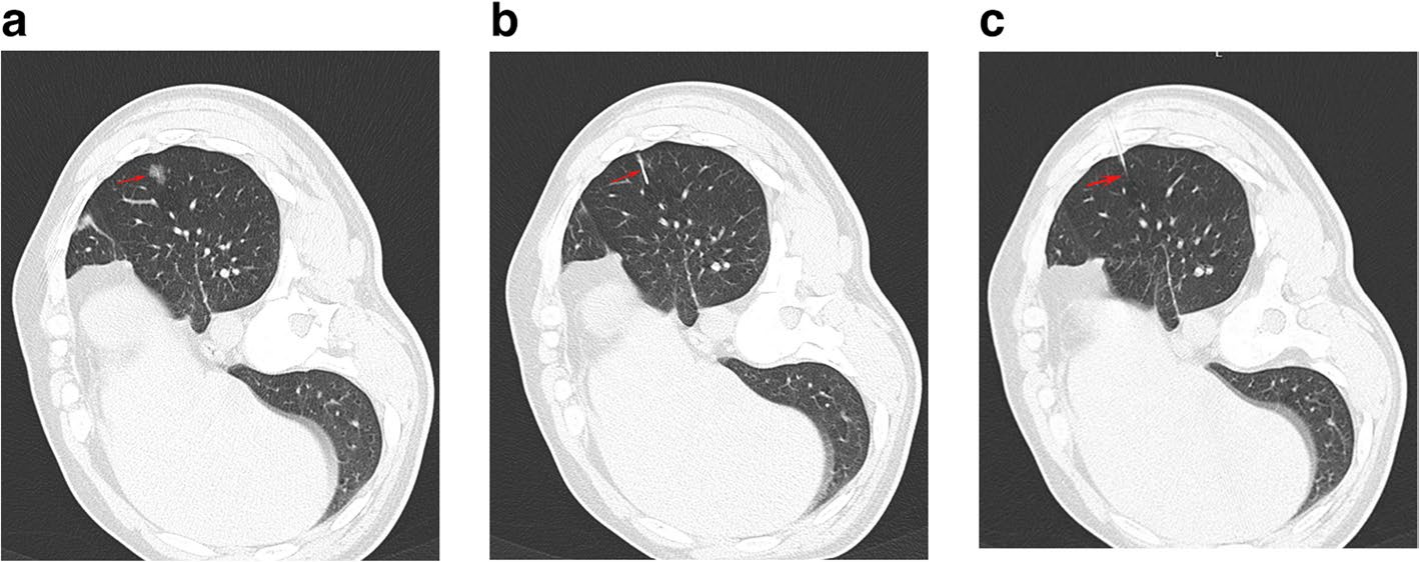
The CT-guided hook-wire GGN localization procedure. a CT images revealing GGN (arrow) at the left lower lobe. b Puncture needle (arrow) positioning near the GGN. c Hook-wire (arrow) positioning via the needle for GGN localization
